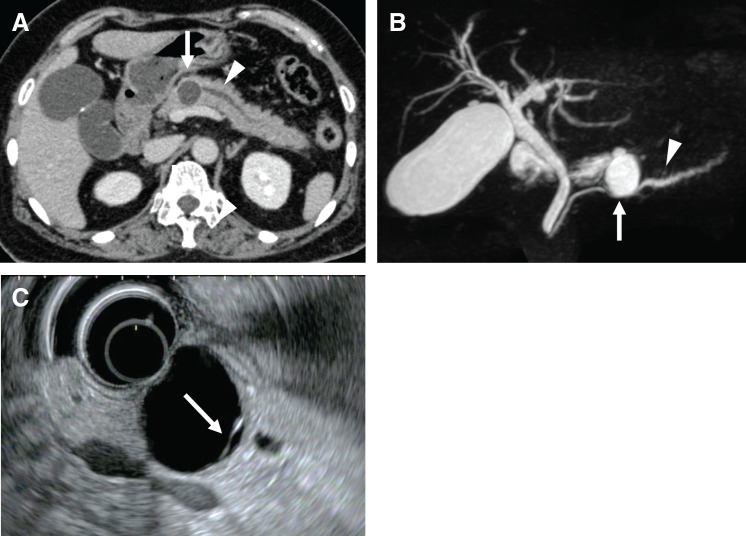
Preoperative imaging. CT axial image (A) and MRCP (B) show a cystic lesion in the pancreatic body (arrow) and dilatation of the main pancreatic duct at its distal end (arrowhead). Endoscopic ultrasound (C) image showing a cystic lesion with internal septations in the pancreatic body (arrow). MRCP, magnetic resonance cholangiopancreatography
